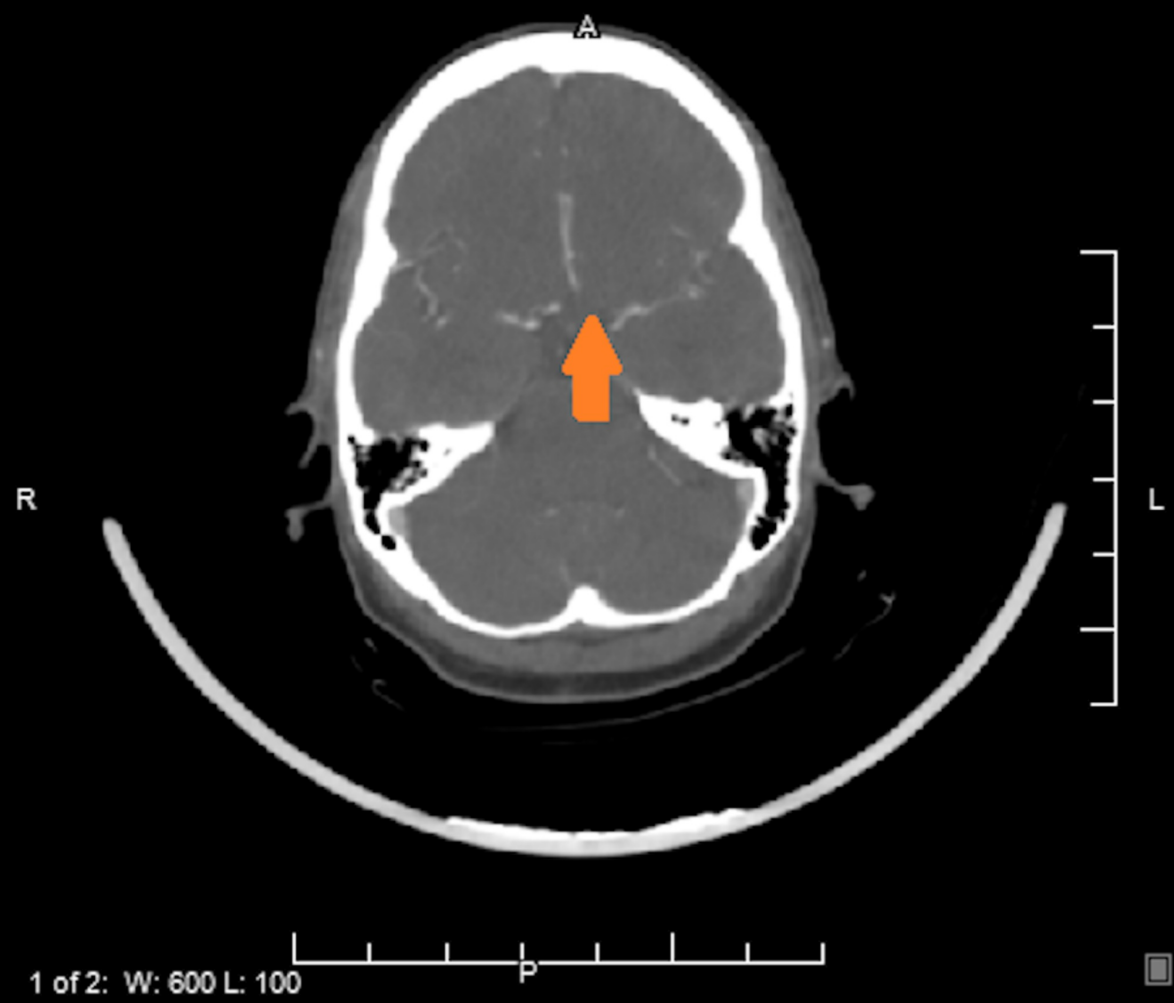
CT angiography of the head and neck: atresia of the A1 segment of the left anterior cerebral artery (orange arrow)
